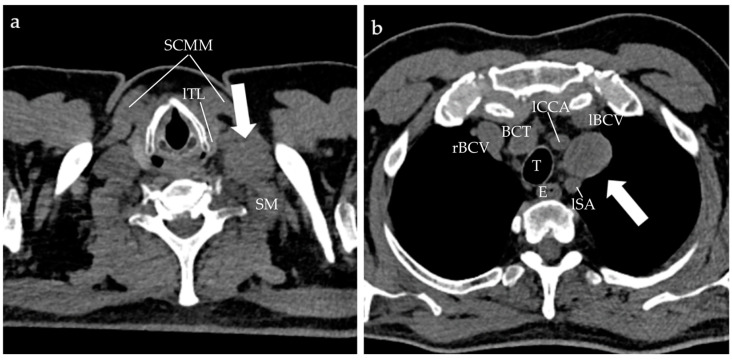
Unenhanced CT scan of the neck (a) and thorax (b), showing left supraclavicular lymphadenopathy (arrow in (a)) and left paratracheal lymphadenopathy (arrow in (b)). SCMM = sternocleidomastoidian muscle; SM = scalenus muscle; lTL = left thyroid lobe; rBCV = right brachiocephalic vein; lBCV = left brachiocephalic vein; BCT = brachiocephalic trunk; lCCA = left common carotid artery; lSA = left subclavian artery; T = trachea; E = esophagus.
